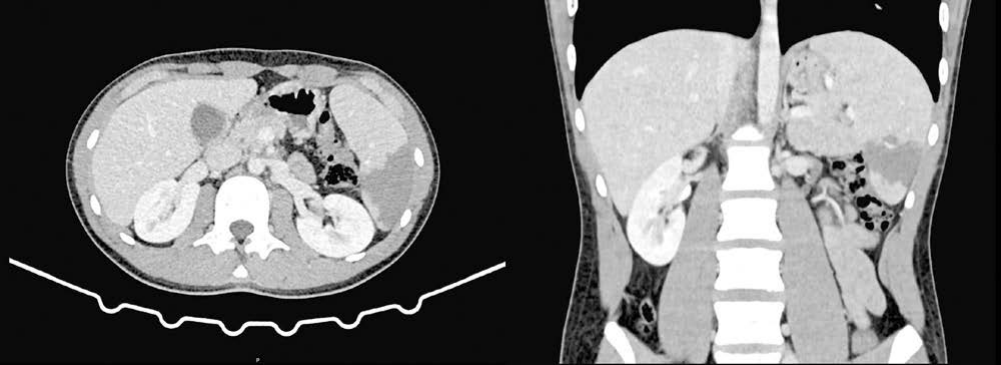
Contrast-enhanced abdominal CT showing a wedge-shaped zone of hypodensity on the spleen tissue.
